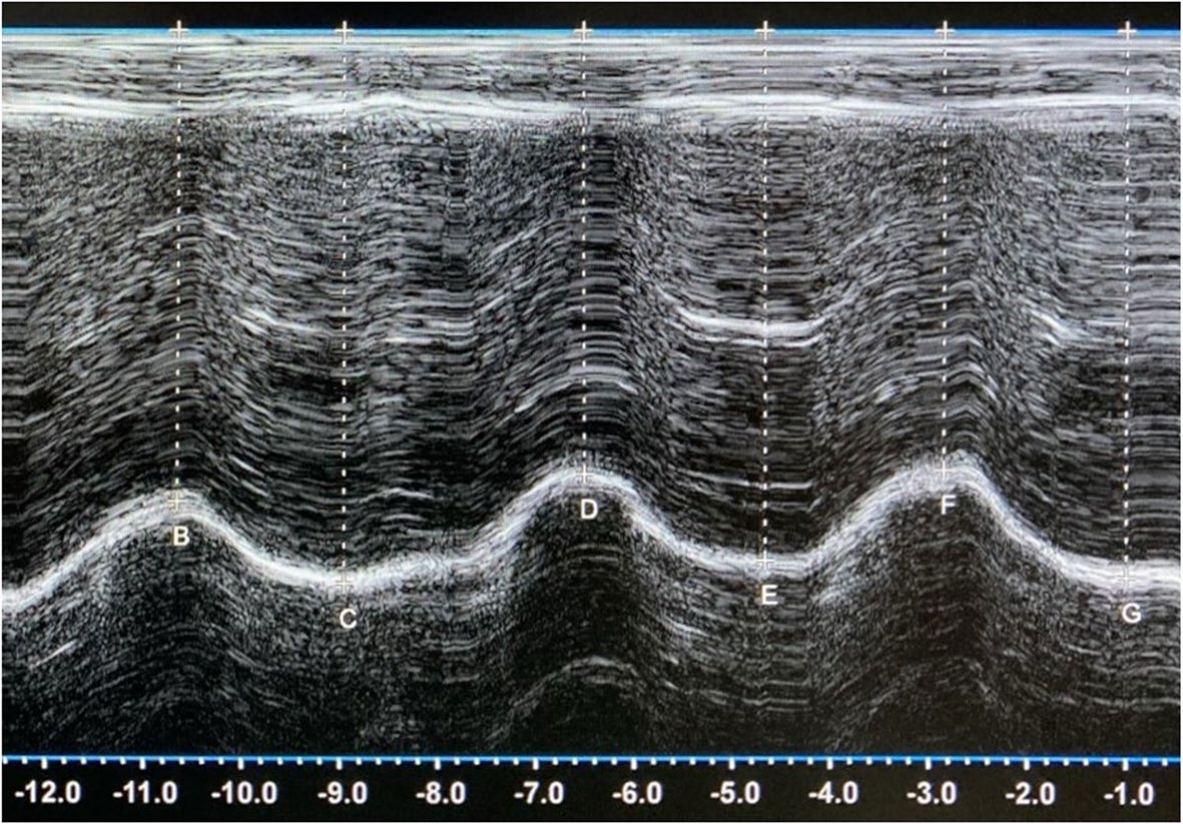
M-mode ultrasonographic image of the diaphragm motion. The cursor is placed on the peak of the curve at the end-inspiratory (upper peaks B, D, F) and end-expiratory (lower peaks C, E, G) phase of breathing with a vertical line to the baseline
